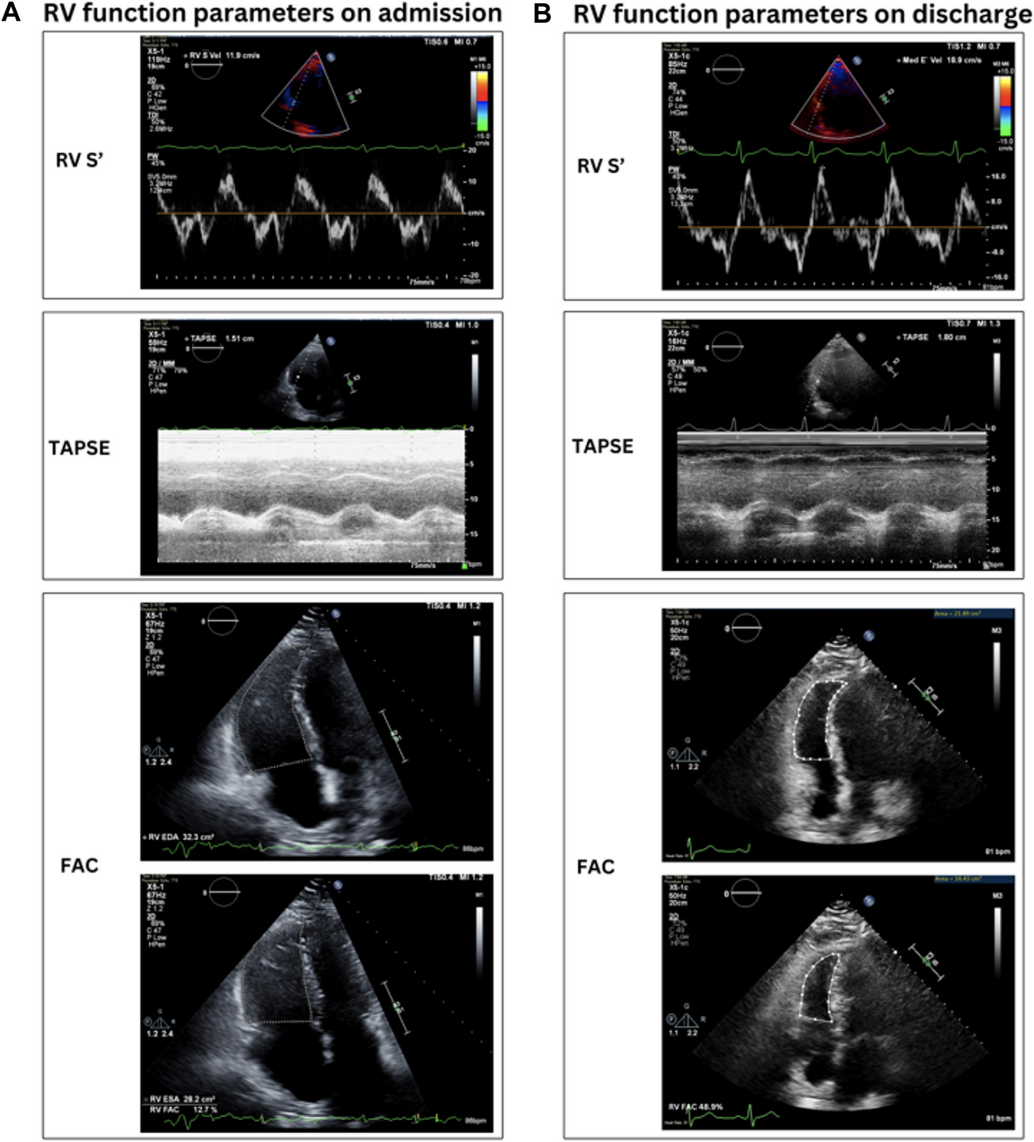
Transthoracic Echocardiogram Showing Right Ventricular Features Before and After Supportive Treatment (A) RV function parameters on admission: RV S′ velocity 11.9 cm/s, TAPSE 1.51, and FAC 12.7%. (B) RV function parameters before discharge: RV S′ velocity 18.9 cm/s, TAPSE 1.8, and FAC 48.9%. FAC = fractional area change; RV = right ventricle; RV S′ = peak lateral tricuspid annular systolic velocity; TAPSE = tricuspid annular plane systolic excursion.
